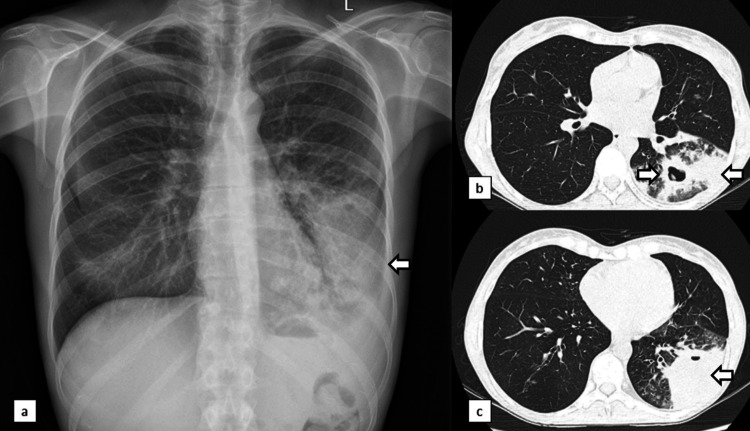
Chest X-rays and CT scan after antibiotic therapy and Voriconazole Chest X-rays (image a) and CT scans (images b and c, axial sections) reveal a complete opacity of the lower lobe of the left lung. CT-guided aspiration was performed, revealing the presence of purulent material. CT, computed tomography
